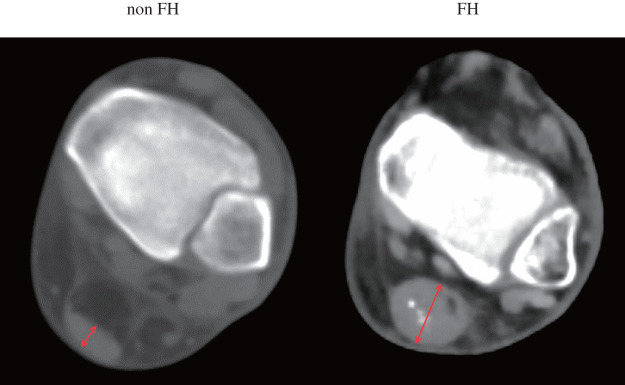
CT imaging of the Achilles tendon in patients with familial and non-FH. The red arrow highlights the measured thickness of the Achilles tendon. FH: familial hypercholesterolemia; CT: computed tomography
